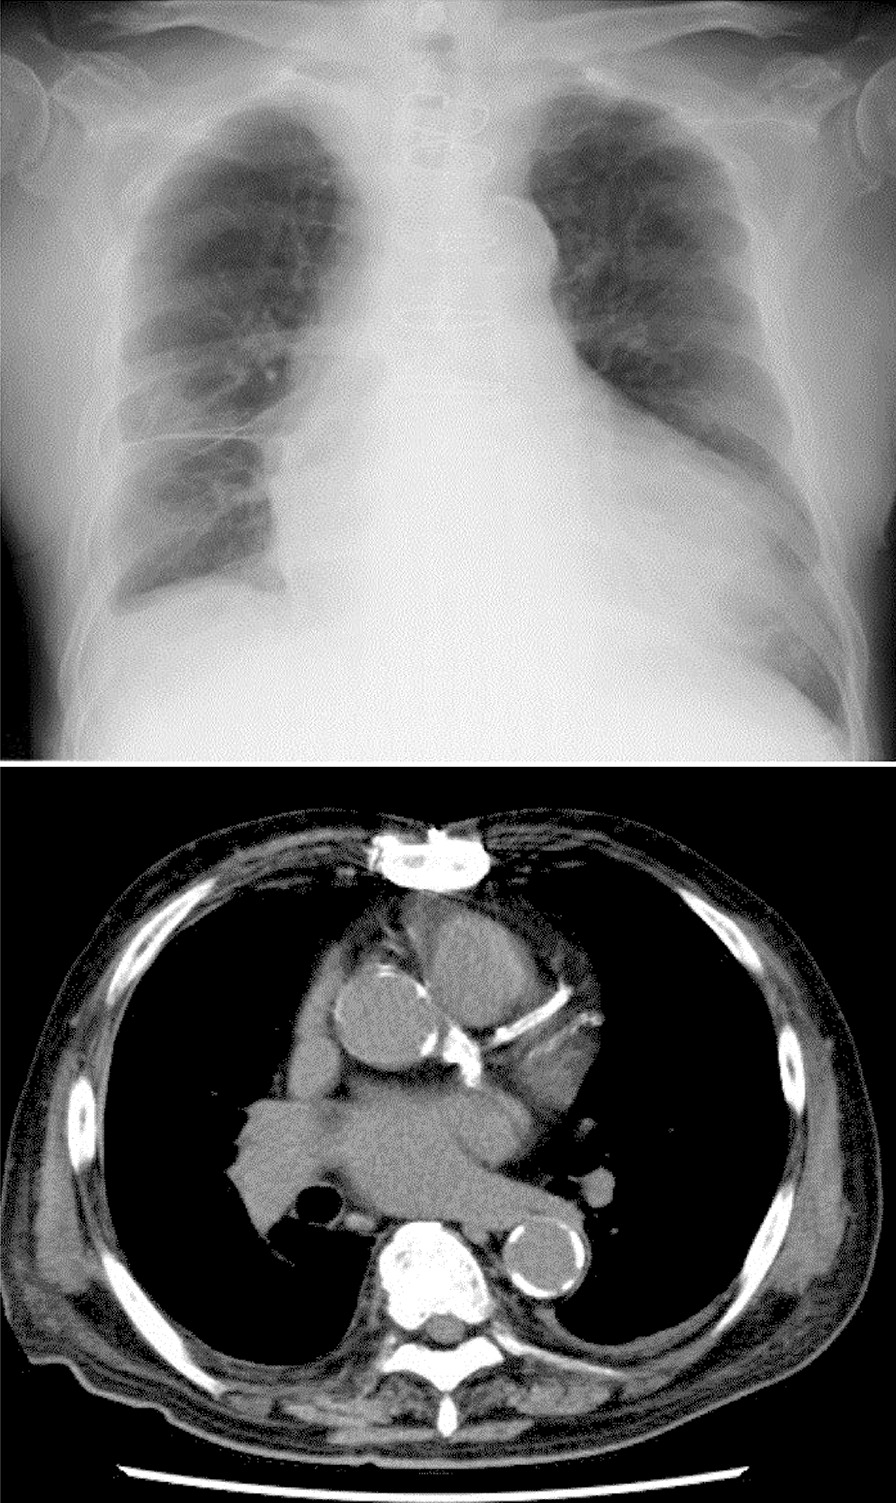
Preoperative chest radiography and computed tomography in Case 2. Chest radiography and computed tomography (CT) showing a CTR of 72% and severely calcified coronary arteries
